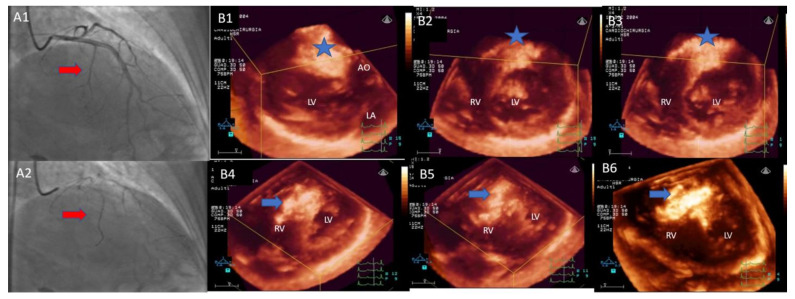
Three-dimensional images of intracoronary myocardial contrast echocardiography. Following contrast agent injection into the first septal branch (A1,A2, red arrow), three-dimensional multiple slice reconstruction from base to cardiac apex (B1–B6) shows myocardial contrast effect in the basal and mid anterior septum (blue star) extending to the moderator band and a large area of the free-wall of the right ventricle (blue arrow). Owing to the high risk of life-threatening extended septal myocardial infarction involving the right ventricle, the patient was considered unsuitable for ASA. AO: aorta; LA: left atrium; LV: left ventricle; RV: right ventricle; ASA: alcohol septal ablation.
